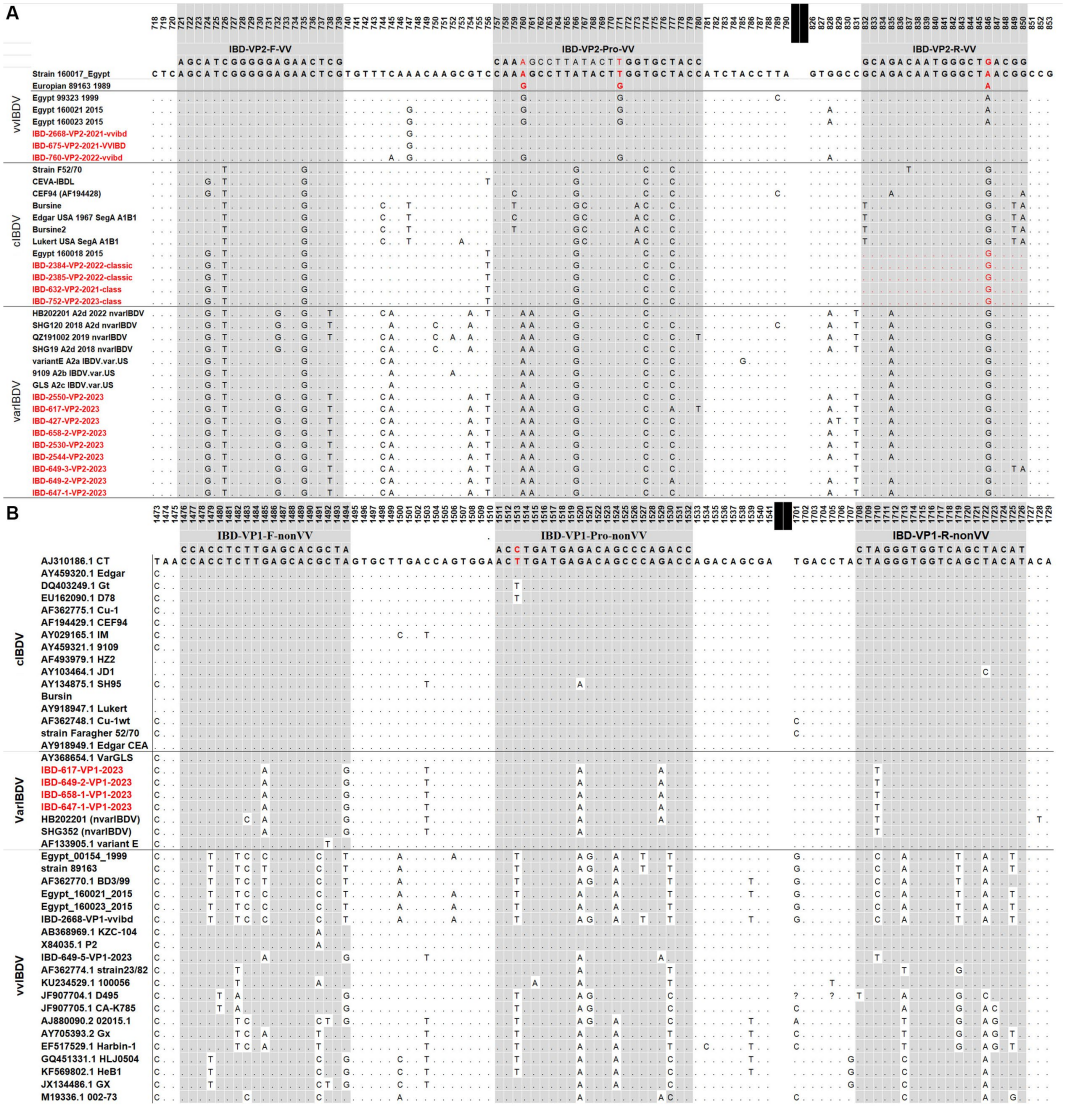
Alignment of forward, reverse, and probe sequences of the real-time RT-PCR. Various representative vvIBDV, classical (cIBDV), and variant (nVarIBDV) strains’ VP2 sequences (A) and VP1 sequences (B). The primers and probes target sites are indicated using the European vvIBDV strain 89163 for VP2 and the classical strain CT for VP1.
